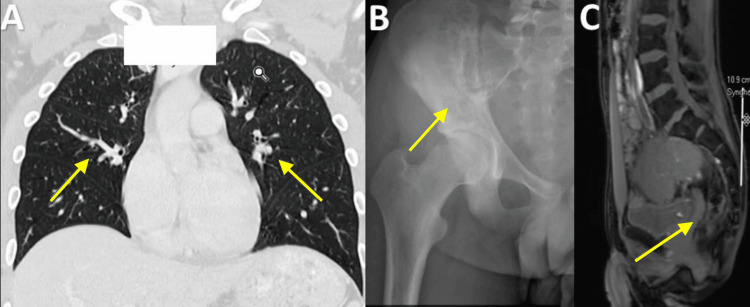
Computed tomography (CT) scan showing lung metastasis (A) and x-ray (B) and CT scan showing bone metastasis in the ischial tuberosity from ES (C).
